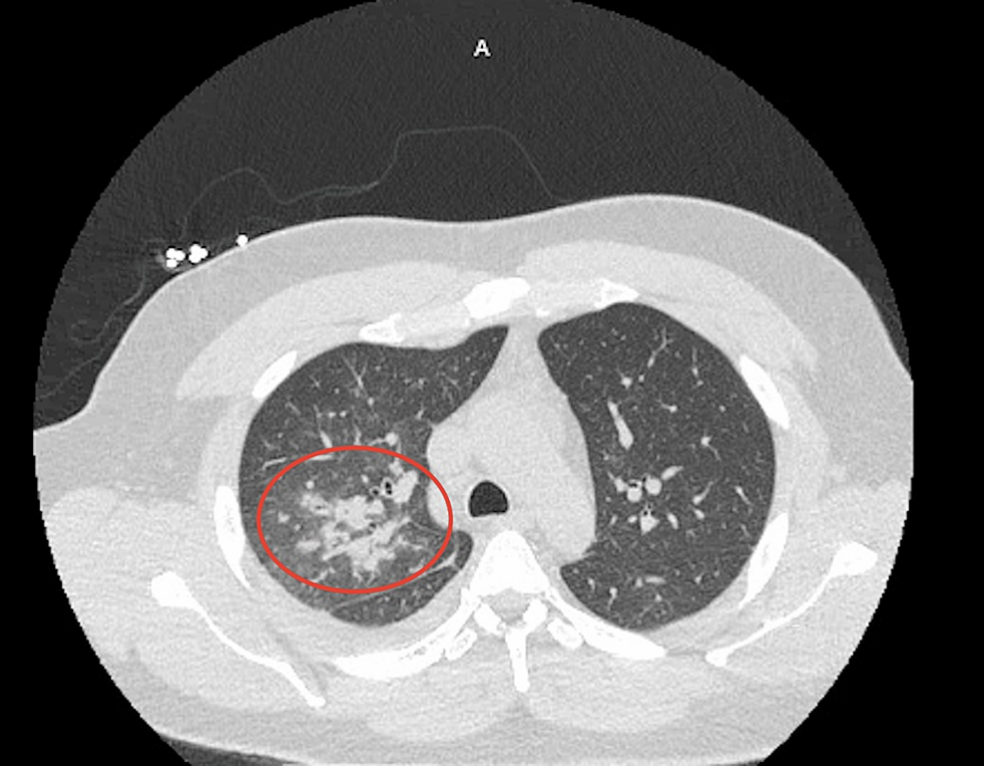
Chest computed tomography (CT) showing multiple nodular lesions in the right lung
